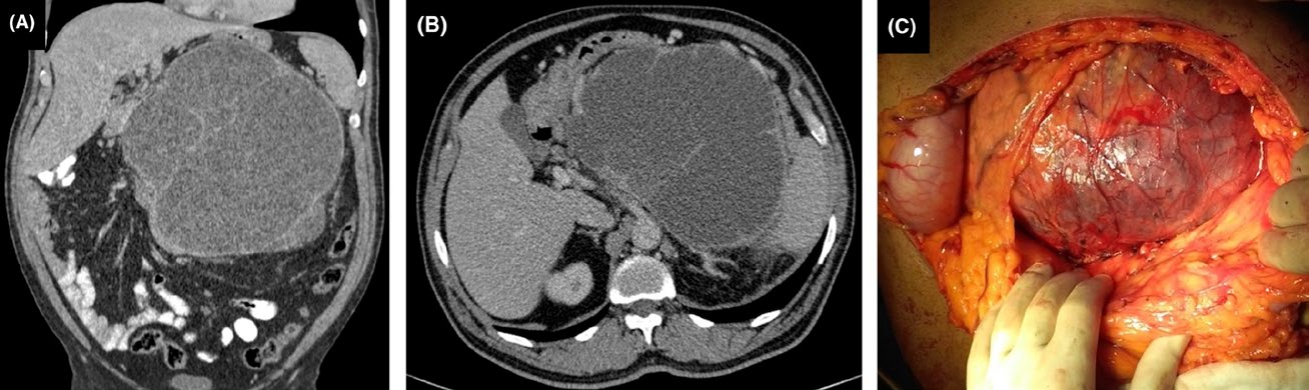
A, CT imaging of the retroperitoneal cystic lesion (coronal view). B, CT imaging of the retroperitoneal cystic lesion (transverse view). C, Intraoperative view of the lesion
